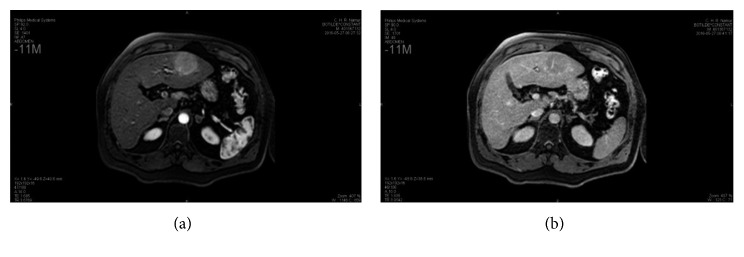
Liver MRI showing the segment III lesion, enhancing at the arterial phase (a) with rapid washout on the portal phase (b).
